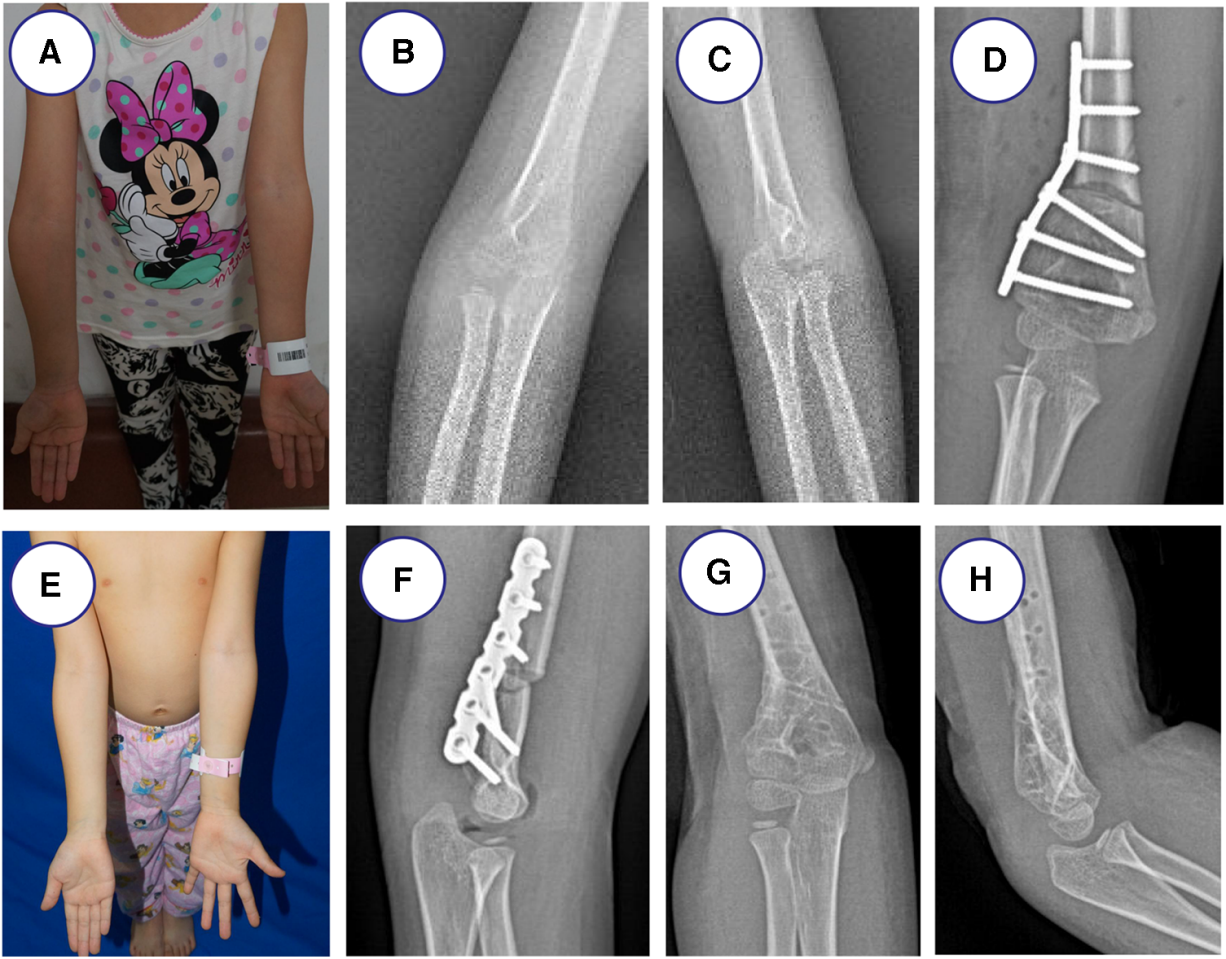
Locking plate fixation of a 9-year-old girl with right cubitus varus owing to right supracondylar humeral fracture. (A) Clinical photograph before surgery. (B,C) Preoperative radiographs showed right cubitus varus before osteotomy. (E) Clinical photograph at the last follow-up time. (D,F) Postoperative radiographs show locking plate fixation after lateral closing-wedge osteotomy. (G,H) Locking plate fixation was removed after 7 months.
